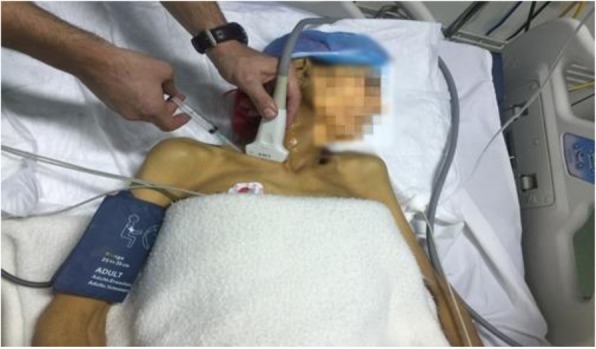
Probe positioning in the supraclavicular fossa during ultrasound guided subclavian vein cannulation via supraclavicular approach
